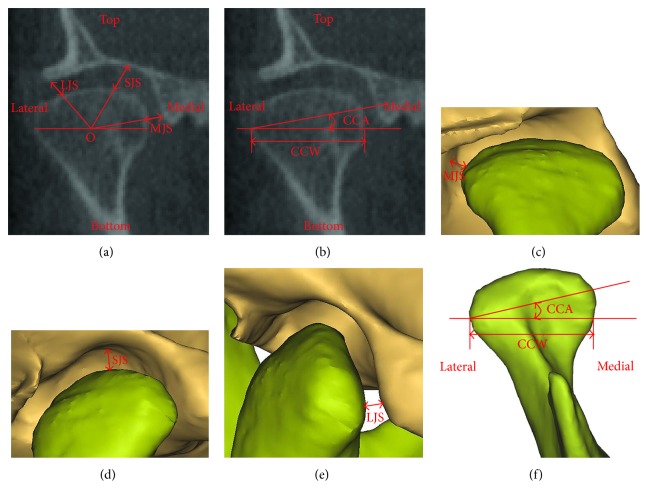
Measurements of the MJS, SJS, LJS, CCA, and CCW in the coronal view: (a and b) on the CBCT images and (c, d, e, and f) in the 3D models. The point O is the midpoint of CCW in part (a). CCA: coronal condylar angle; CCW: coronal condylar width; MJS: medial joint space; LJS: lateral joint space; SJS: superior joint space.
